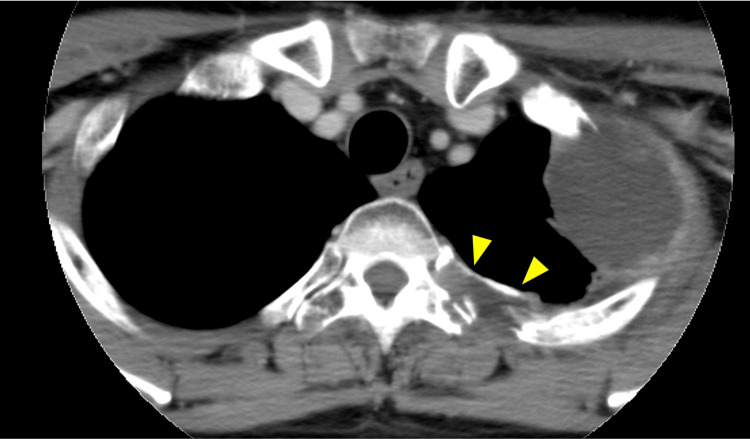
Image findings exacerbated distant metastasis CT showing exacerbation of the left third rib (arrow head) and lung metastasis with significant tumor enlargement in February 2021.
